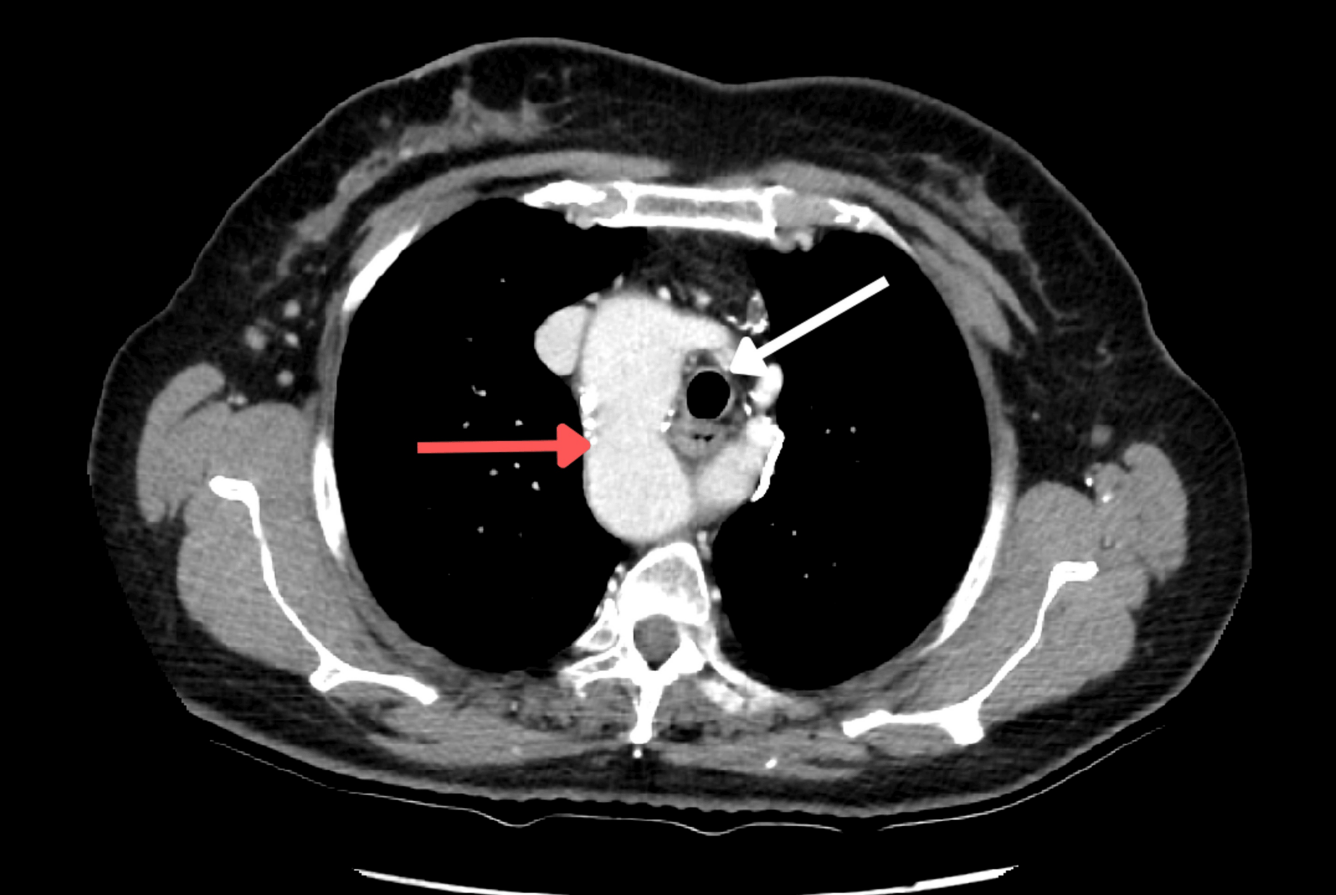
Axial view of a CT scan showing the right aortic arch (red arrow) to the right of the trachea (white arrow)
